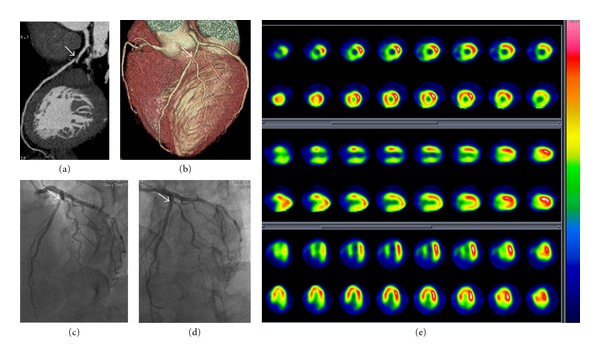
Patient with stenosis in the proximal segment of LAD classified as ≥50% on CTCA related with large and reversible perfusion defects (ischemia) in apex, anterior, and septal wall on MPI. (a) and (b) Multiple reformation (MPR) and maximal intensity projection (MIP) on CTCA showed noncalcification plaque in the proximal segment of LAD adjacent to the ostia of diagonal branch causing a significant stenosis (arrows), and no positive results on other main arteries were found. (c) ICA of left coronary arteries showed severe stenosis (arrow) in the same segment of LAD compared with CTCA. (d) ICA of left coronary arteries showed successful stenting (arrow) for the lesion in the proximal segment of LAD. (e) SPECT MPI study at stress (odd row) and rest (even row) showed large and reversible perfusion defects in the area of apex, anterior and septal wall corresponding to the territory of LAD, indicating large and severe myocardial ischemia.
